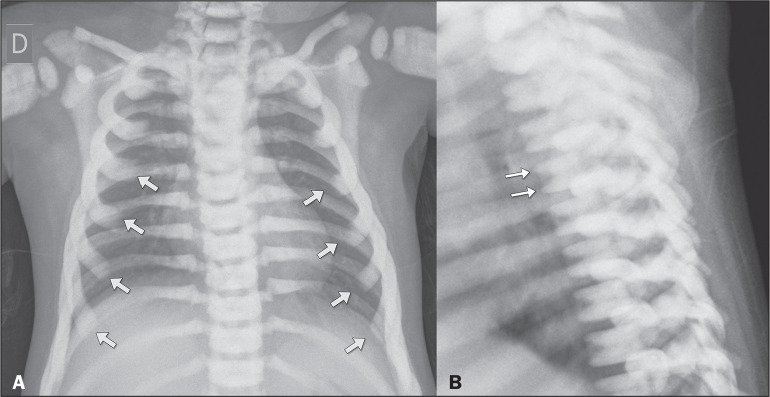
Chest X-rays of a 16-monthold patient with the autosomal recessive
